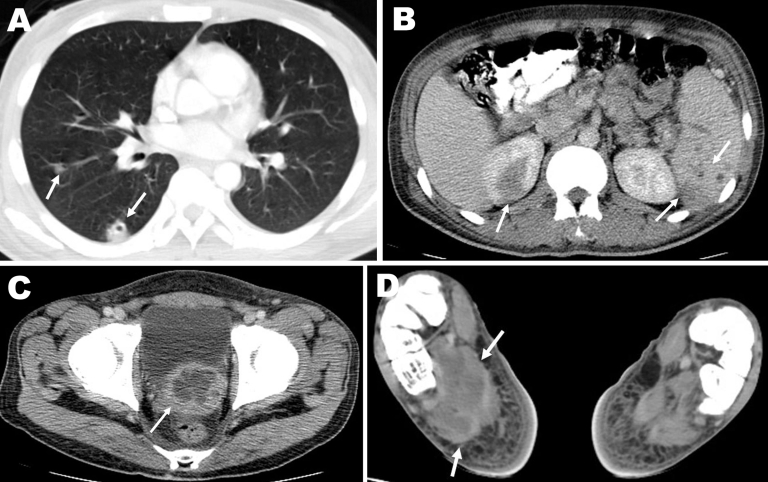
Computed tomography images showing multiple abscesses in the right lung (A), spleen and upper pole of the right kidney (B), prostate gland (C), and plantar aspect of the right foot (D) (arrows) of the patient.
